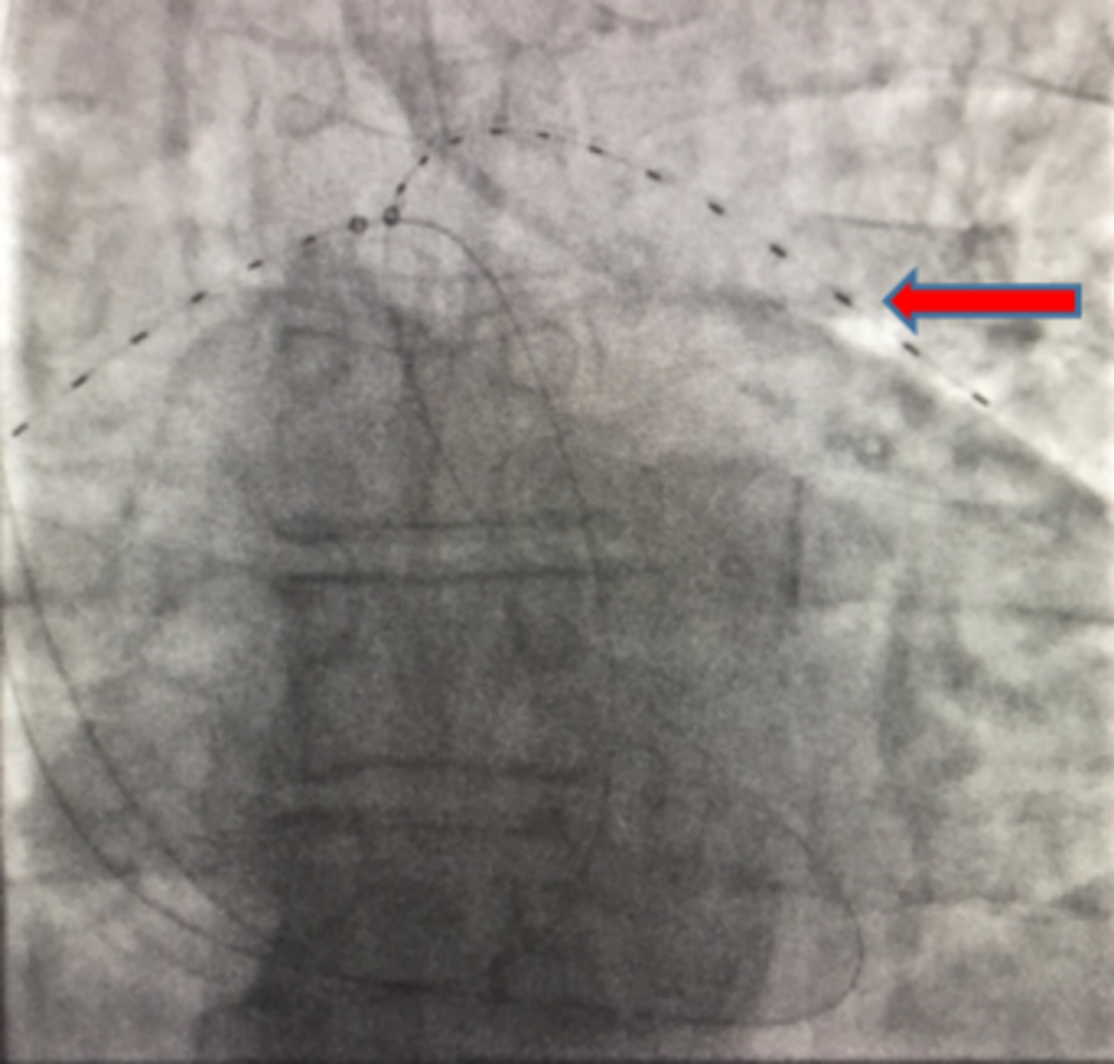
Bilateral EKOS catheters on fluoroscopy. Each marker is 1 cm (red arrow) and indicates an area of ultrasound.
